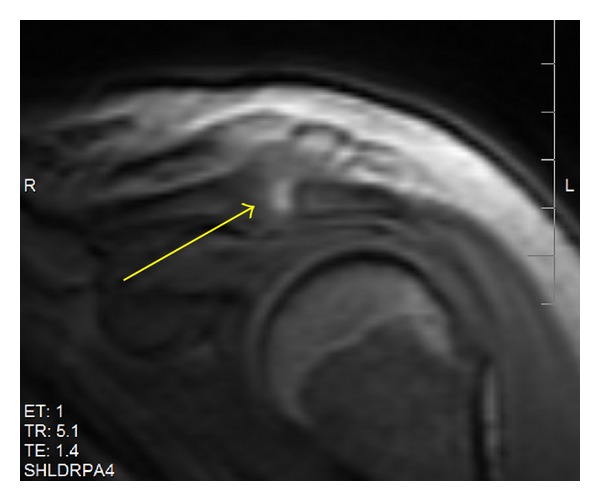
Left shoulder magnetic resonance imaging revealing edema within the acromioclavicular (AC) joint and edema and fluid outside of the joint. There is distal clavicular cortical destruction (arrow).
